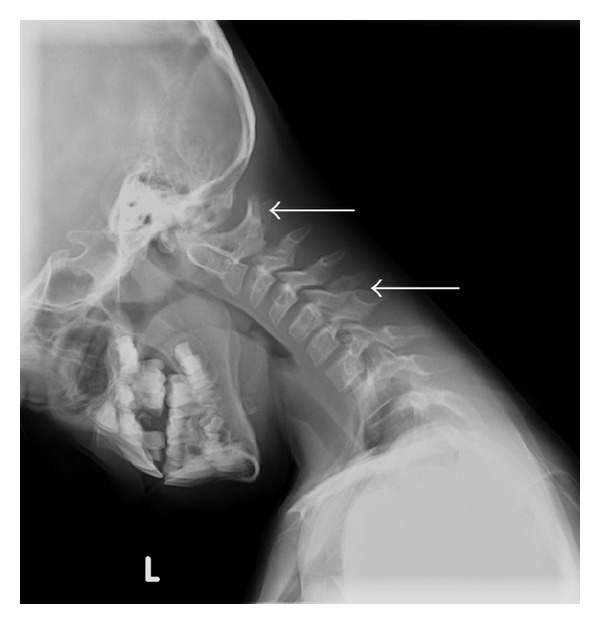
Radiograph depicting the Klippel-Feil anomaly with partial fusion of the posterior elements of C1-2 and C5-6 (arrows). Note also that there are 8 cervical vertebrae.
